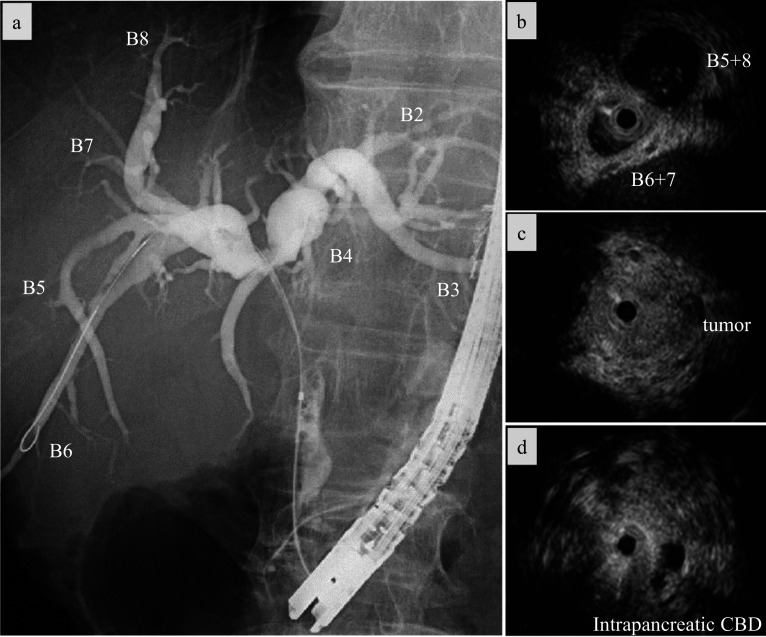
Assessment of bile duct. a Endoscopic retrograde cholangiopancreatography showed stenosis of the common hepatic duct. b − d Intraductal ultrasonography revealed bile duct wall-thickening from just below the B4 bifurcation and at the anterior–posterior bifurcation to the intrapancreatic common bile duct (CBD). B5 + 8, right anterior bile duct; B6 + 7, right posterior bile duct
